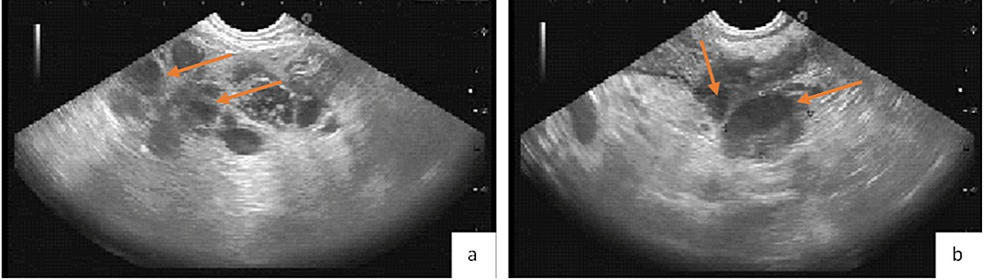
Endoscopic Ultrasound (EUS) Demonstrating a Large Cluster/of Honey Combing Lesions Near the Pancreatic Head (a) and a Matted Cluster of Hypoechoic Lesions Near the Gastric Antrum (b).
